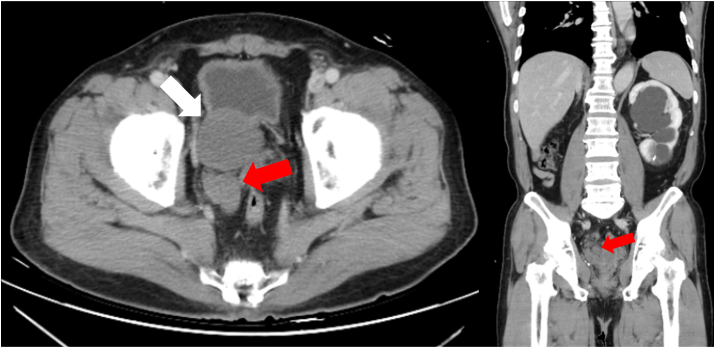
Axial and coronal CT images revealed a 48-mm right seminal vesicle cyst (white arrow) with a dilated right vas deferens (red arrow), which may indicate obstruction of the right ejaculatory duct.
